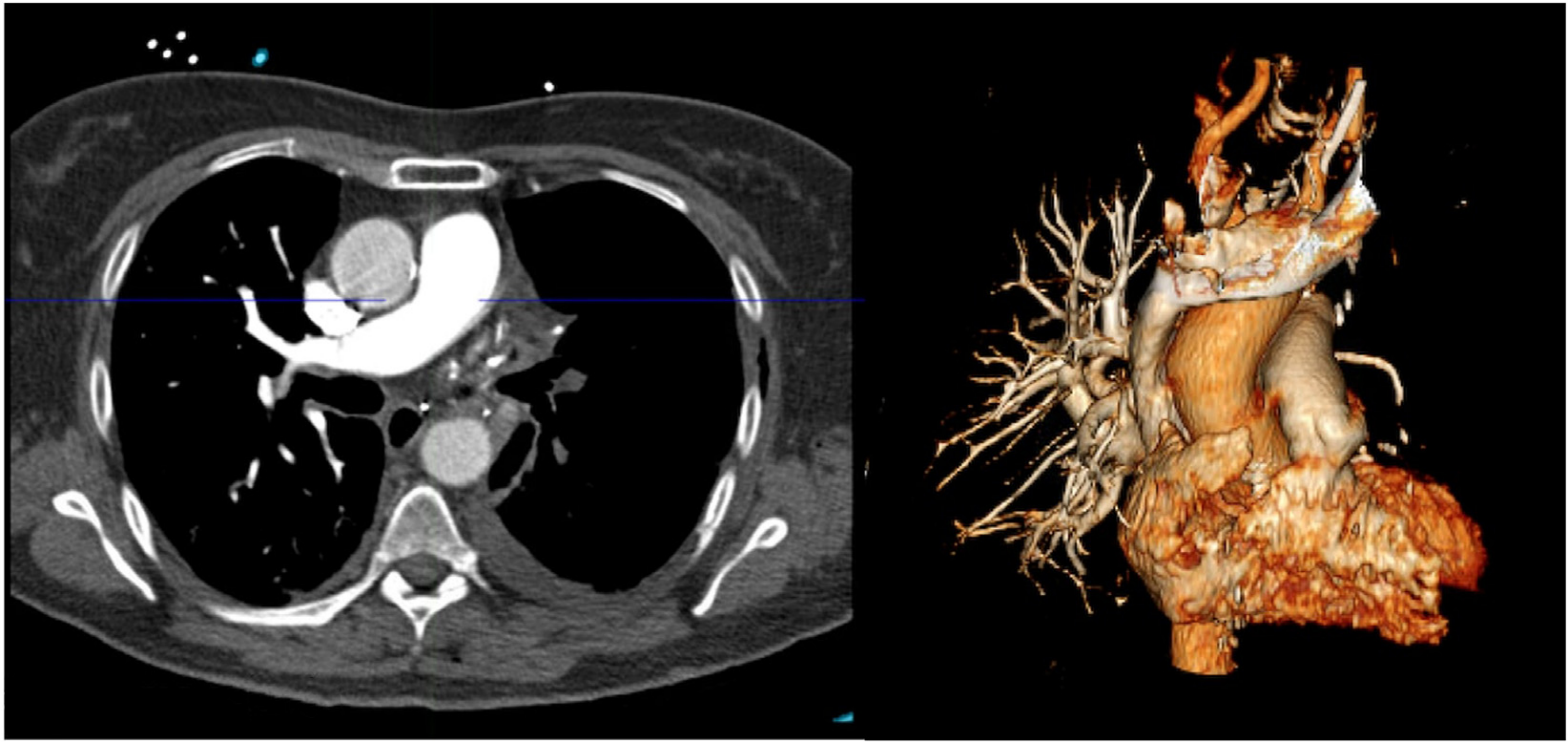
Thoracic Computed Tomography Angiography Revealing Acute Left Pulmonary Embolism
